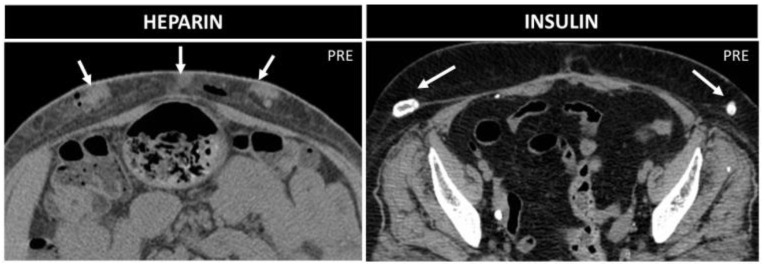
Heparin vs. insulin treatment nodules in two 70-year-old patients. Axial not-enhanced CT in the first patient shows some collections with parenchymatous density, which indicate bleeding, and small air bubbles. In the second patient, instead, lipodystrophies with peripheral calcifications are seen.
